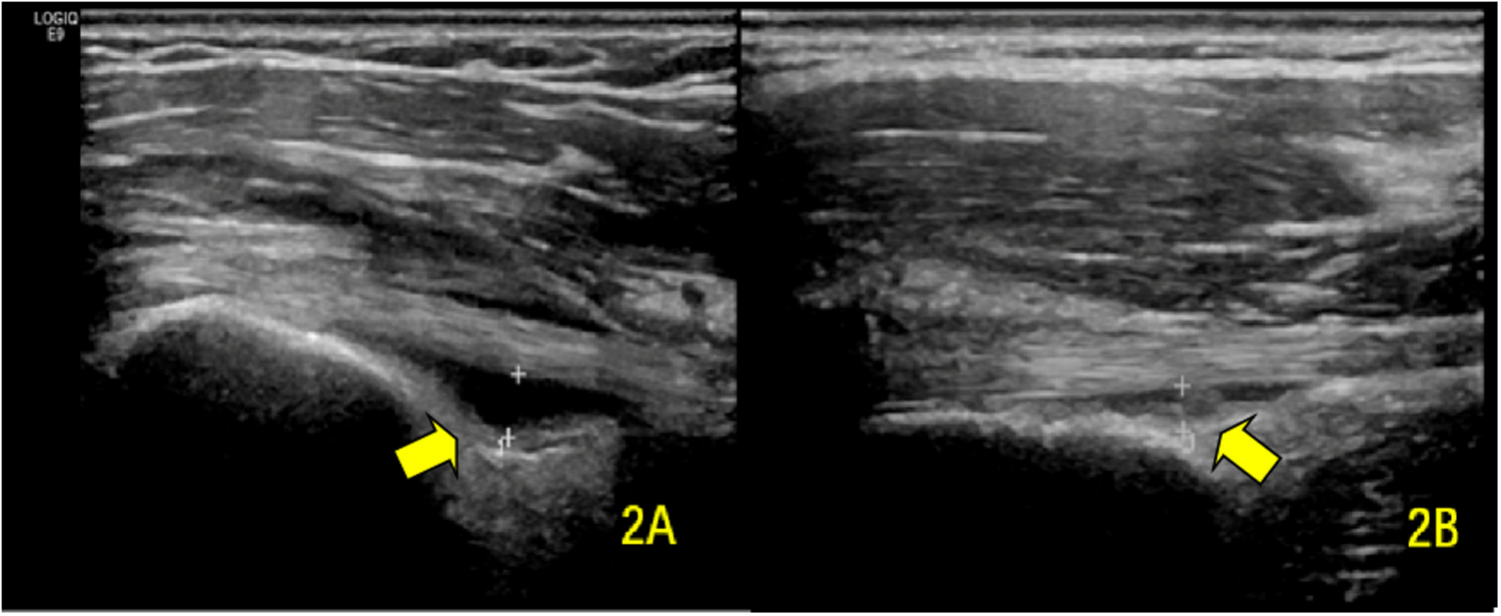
Transverse ultrasound images showing BICT sheath effusion (A) pre-rehabilitation and (B) post-rehabilitation. The arrows demarcate the fluid-distended BICT synovial sheath.
